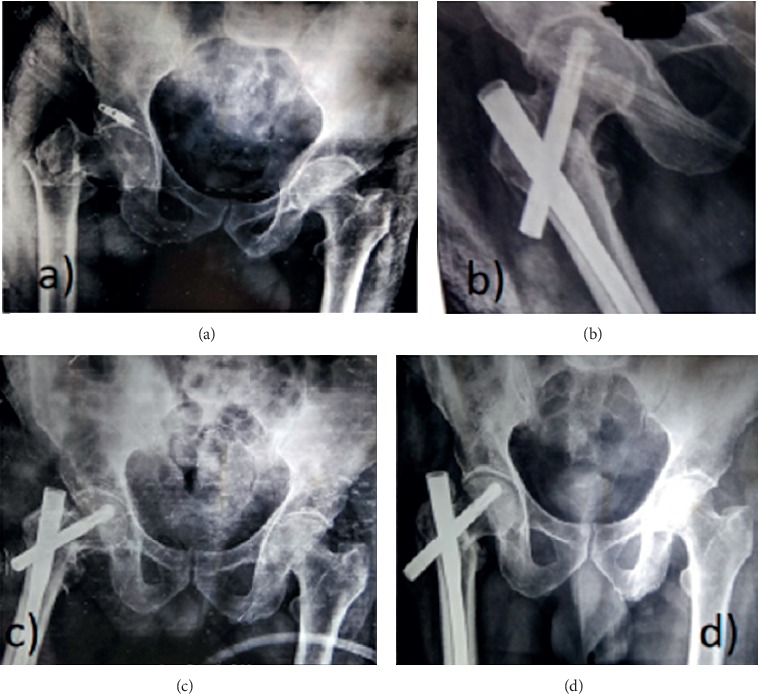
(a) Preoperative X-ray. (b) Immediate postoperative X-ray with lateral entry showing varus reduction, (c) at 6 weeks and (d) at final follow-up showing screw back out.
